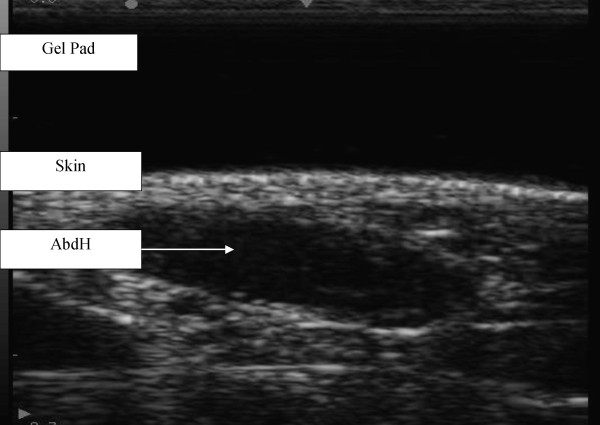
Ultrasound image of abductor hallucis muscle from Chison 8300 (longitudinal view).
